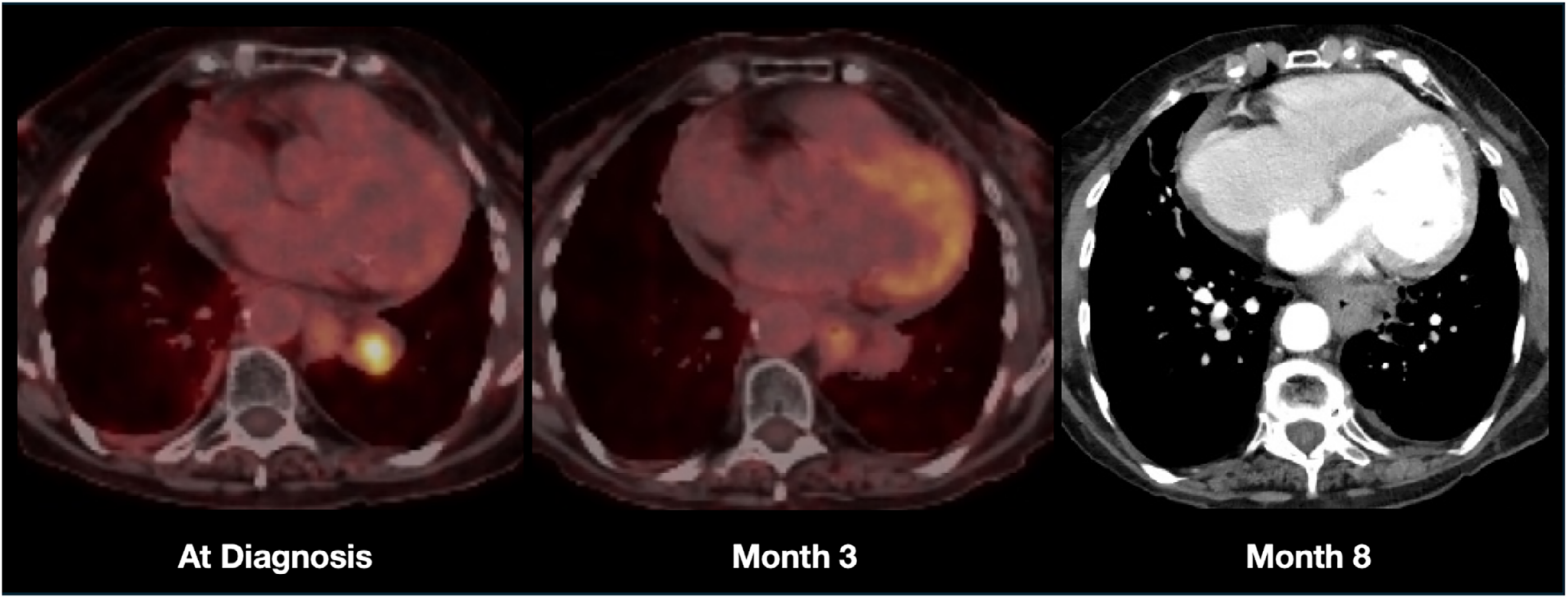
PET/CT at diagnosis and 3 months and CT at 8 months follow‐up of a 78‐year‐old female with metastatic non‐small cell lung cancer (large cell subtype) treated for a centrally located oligometastasis using the Synchrony LWR technique (tumor diameter: 28 mm; GTV: 6.4 cc; PTV: 14.6 cc; 56 Gy/8 fractions).
